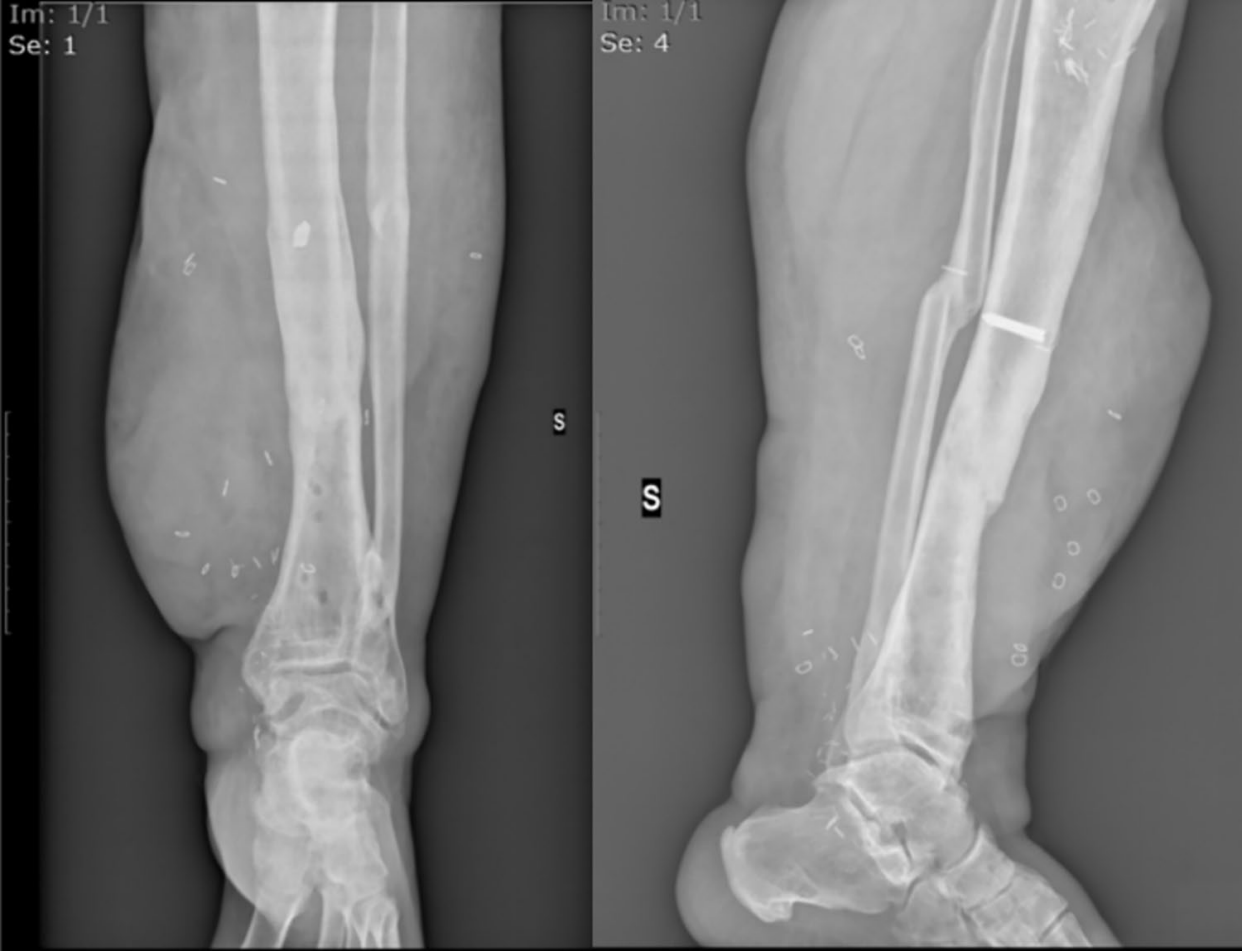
Radiographs post external fixator removal with evident bone consolidation
